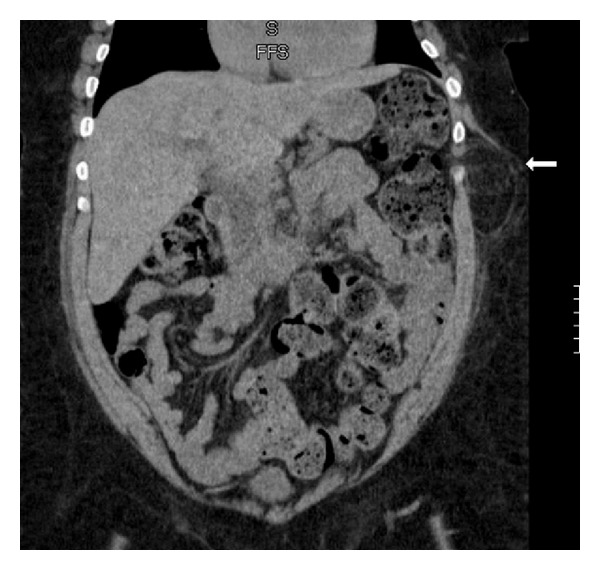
Coronal CT view: intercostal hernia between 10th rib and 11th rib at left midaxillary line (white arrow).
